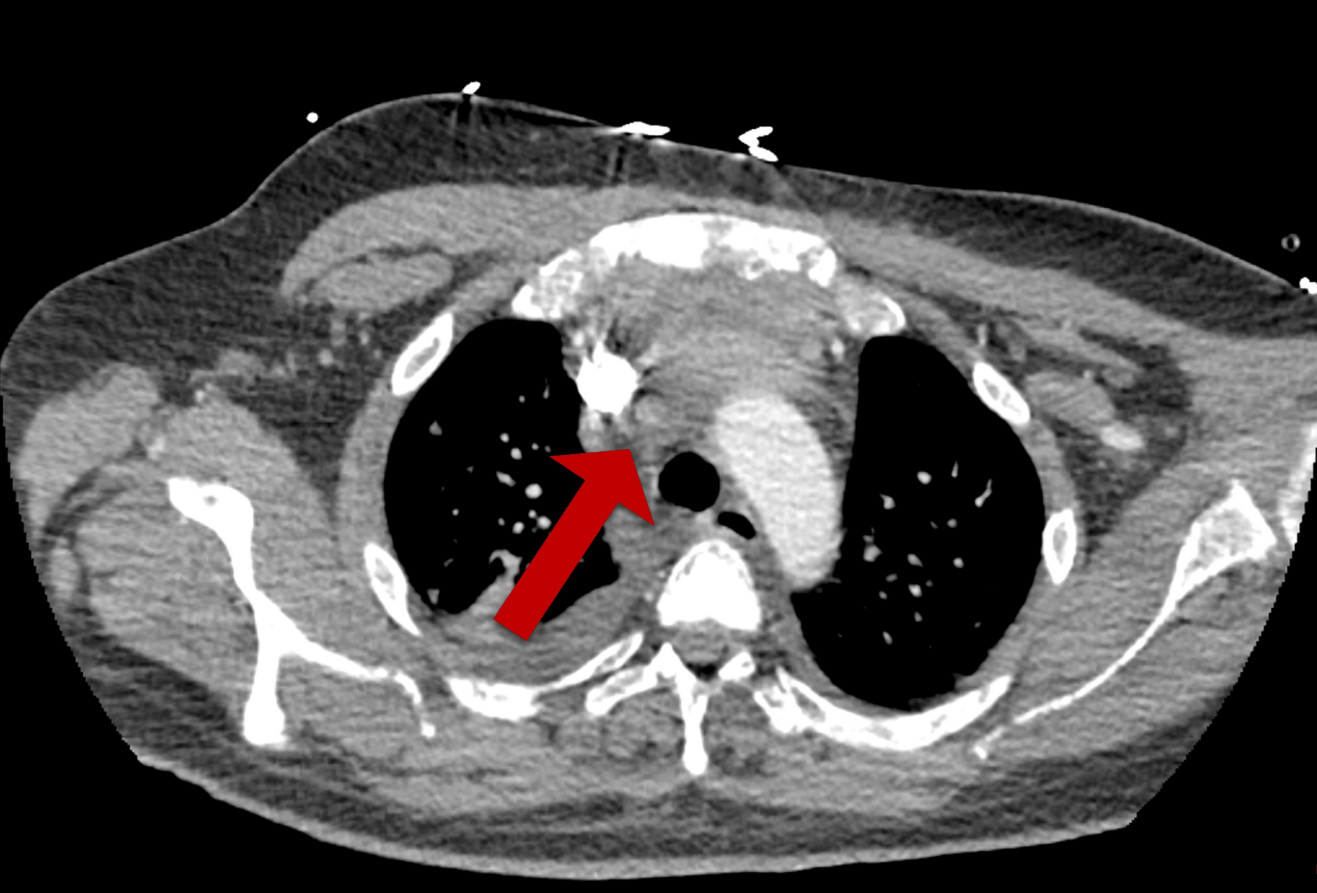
Stable mediastinal hematoma on the CT scan (arrow)
